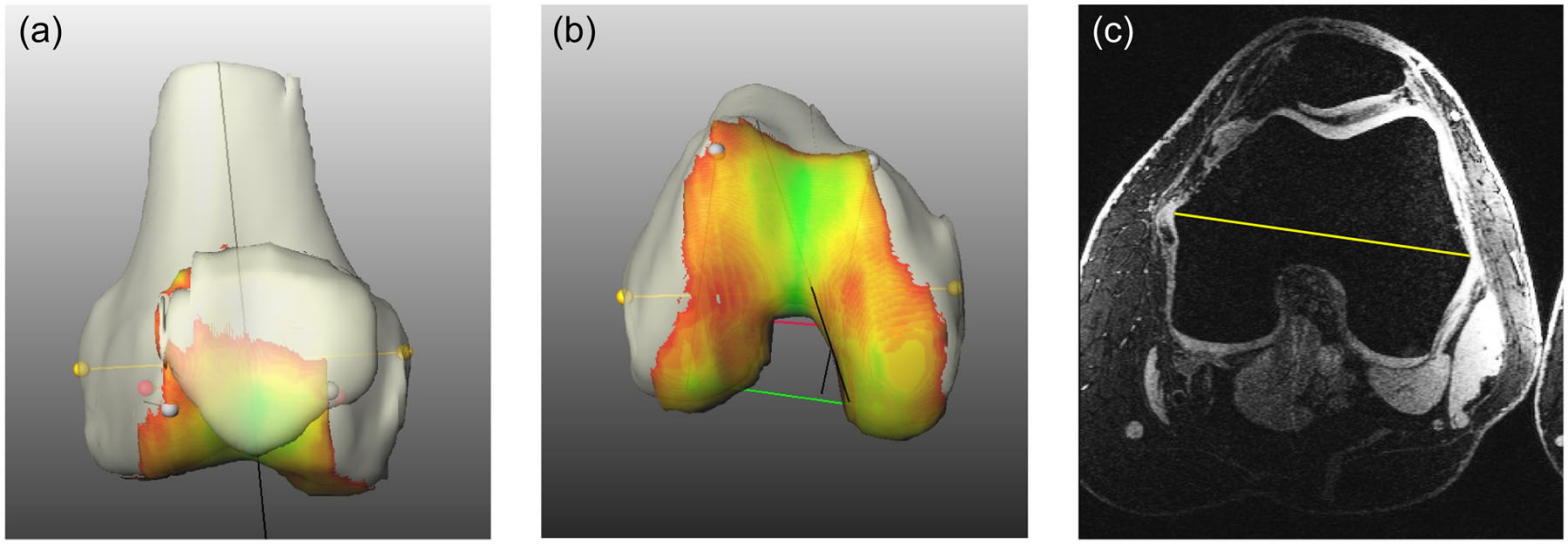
Three‐dimensional reconstruction of a right femur. The bone is beige, thinner cartilage is red, and thick cartilage is green. The yellow line marks the transepicondylar distance. (a) Anterior‐posterior view of the distal femur and patella. (b) Inferior view of the femur cartilage area. (c) Axial MRI view of the distal femur for manually measuring the TEA distance (yellow line).
